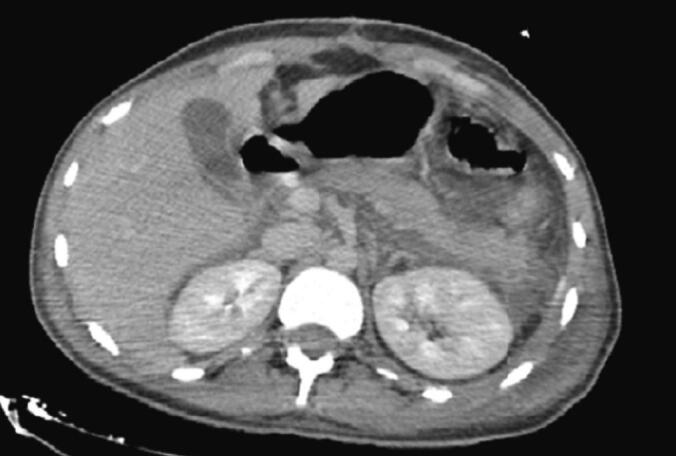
Axial CT section at day 3 showing post-traumatic acute pancreatitis.
